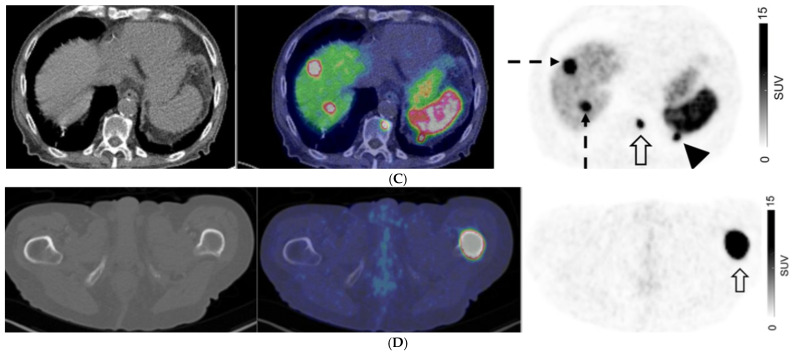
A 76-year-old man with well-differentiated G3 pancreatic neuroendocrine tumor (SUVmax 131.5, Krenning score 4), with bilobar liver metastases on CT. ^68^Ga-DOTATATE PET (A) MIP image; (B–D) Axial images: CT (left), fused PET/CT (middle), and PET (right). PET shows an intensely-avid locally advanced pancreatic tail mass (SUVmax = 132; solid arrow in (B)), liver metastases (biopsy proven; dotted arrows in (B,C)), peritoneal deposit (arrowhead in (C)) and bone metastases (open arrows in (C,D)).
